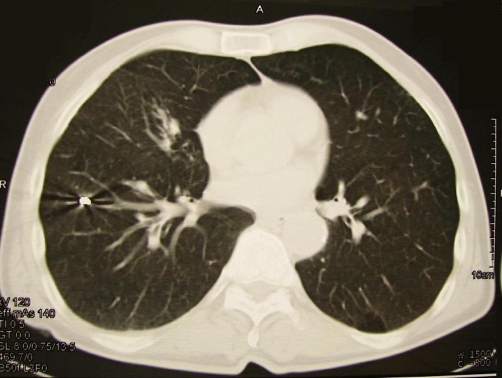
A chest CT-scan that performed, presented the Steinman wire to be located in the superior segment of the right lower lobe.
